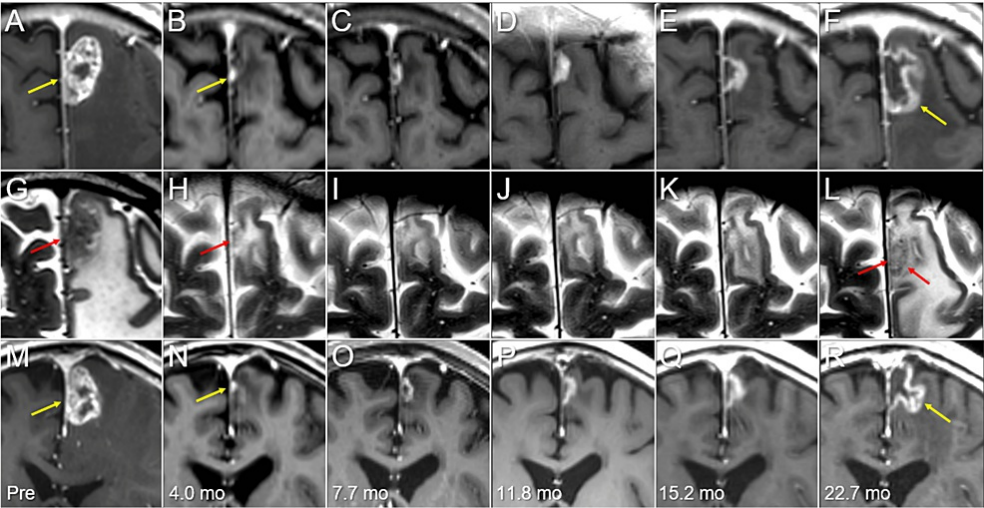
Magnetic resonance images before and after five-fraction stereotactic radiosurgery The images show axial CE-T1-WIs (A-F); axial T2-WIs (G-L); coronal CE-T1-WIs (M-R); 3 days before (pre) SRS (A, G, M); at four months (mo) after SRS (B, H, N); at 7.7 months (C, I, O); at 11.8 months (D, J, P); at 15.2 months (E, K, Q); and at 22.7 months (F, L, R). (A-R) These images are shown at the same magnification and coordinates under co-registration and fusion. (B, H, N) The BM (arrows in A, G, and M) showed remarkable shrinkage at four months, with the tumor remnant being seen as a tiny lesion (arrows in B, H, and N). (C-F, I-L, O-R) The tiny enhancing lesion gradually increased from 7.7 months to 22.7 months. Although no corresponding mass lesion was observed on T2-WI until 15.2 months, i.e., T1/T2 mismatch, the irregularly-shaped mass lesion (arrows in L) appeared at 22.7 months, which was still much smaller than the enhancing lesion (arrows in F, R) (T1/T2 mismatch). CE: contrast-enhanced; WI: weighted image; SRS: stereotactic radiosurgery; BM: brain metastasis
